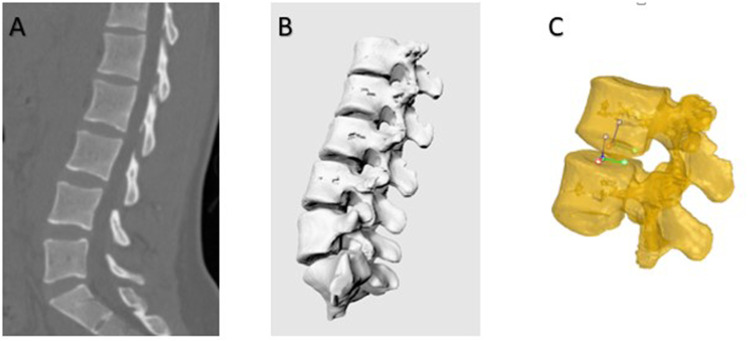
(A) A typical CT scan of a human lumbar spine in the sagittal plane. (B) Three-dimensional anatomic vertebral model constructed from computed tomography. (C) Anatomic coordinate systems were established at the endplates to measure the kinematics of the vertebrae.
